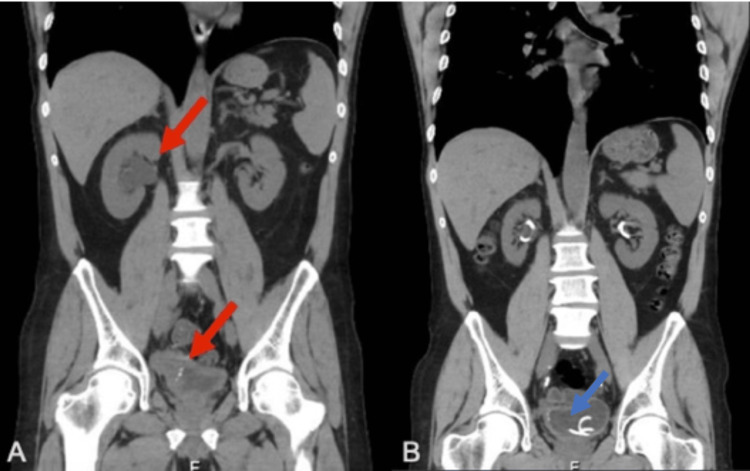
Imaging findings before and during the treatment with nivolumab. A: CT scan showing a large bladder tumor and right hydronephrosis and hydroureter resulting from the extrinsic compression of the right ureter by metastatic pelvic lymph nodes and tumor infiltration of the right ureteral orifice (red arrows). B: Complete bladder response during the treatment with nivolumab (blue arrow). CT = computed tomography
